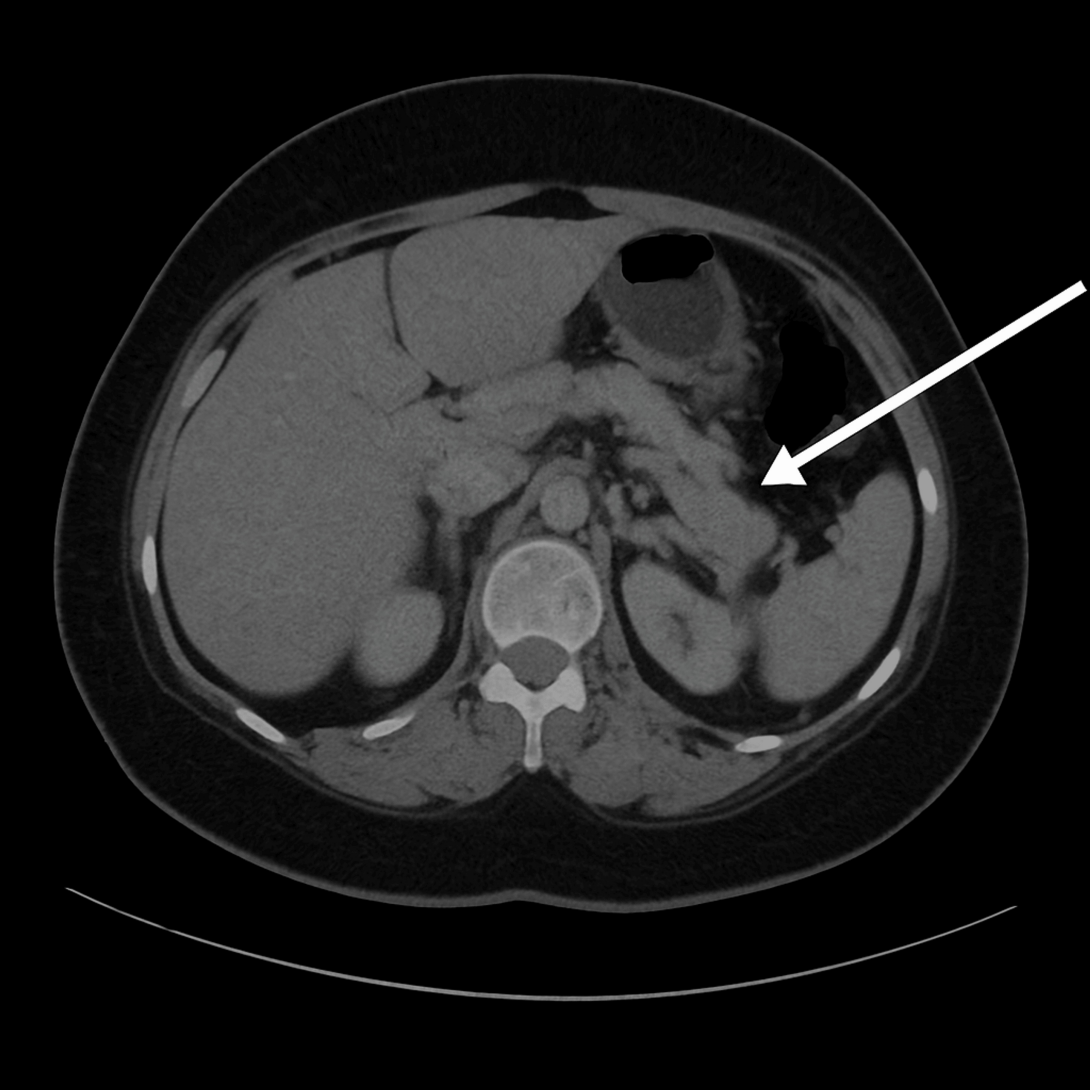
Contrast-enhanced computed tomography scan of the abdomen showing diffuse peripancreatic inflammation. The white arrow highlights the area of fat stranding. Source: Radiology department, Port of Spain General Hospital
